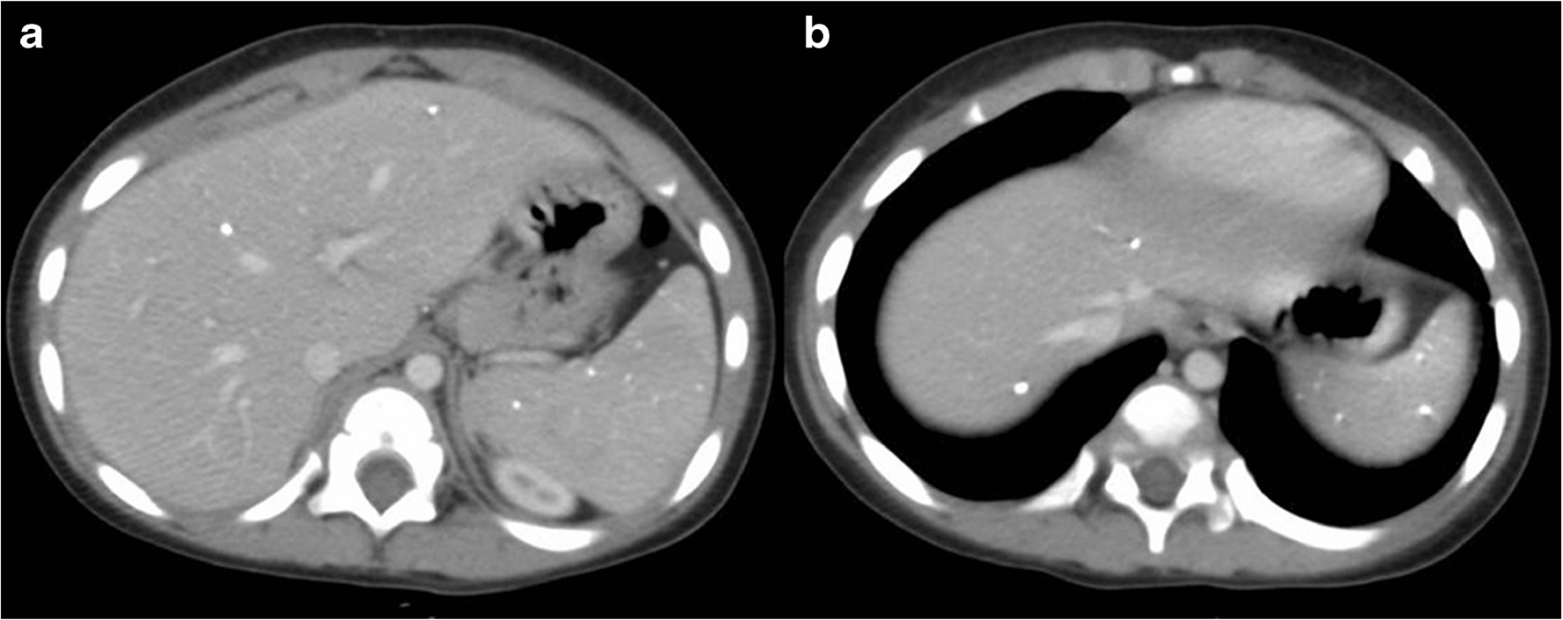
A middle-aged woman with a history of treated tuberculosis. a, b Axial contrast-enhanced CT images reveal multiple calcified lesions in the liver and spleen in keeping with calcified granulomas
